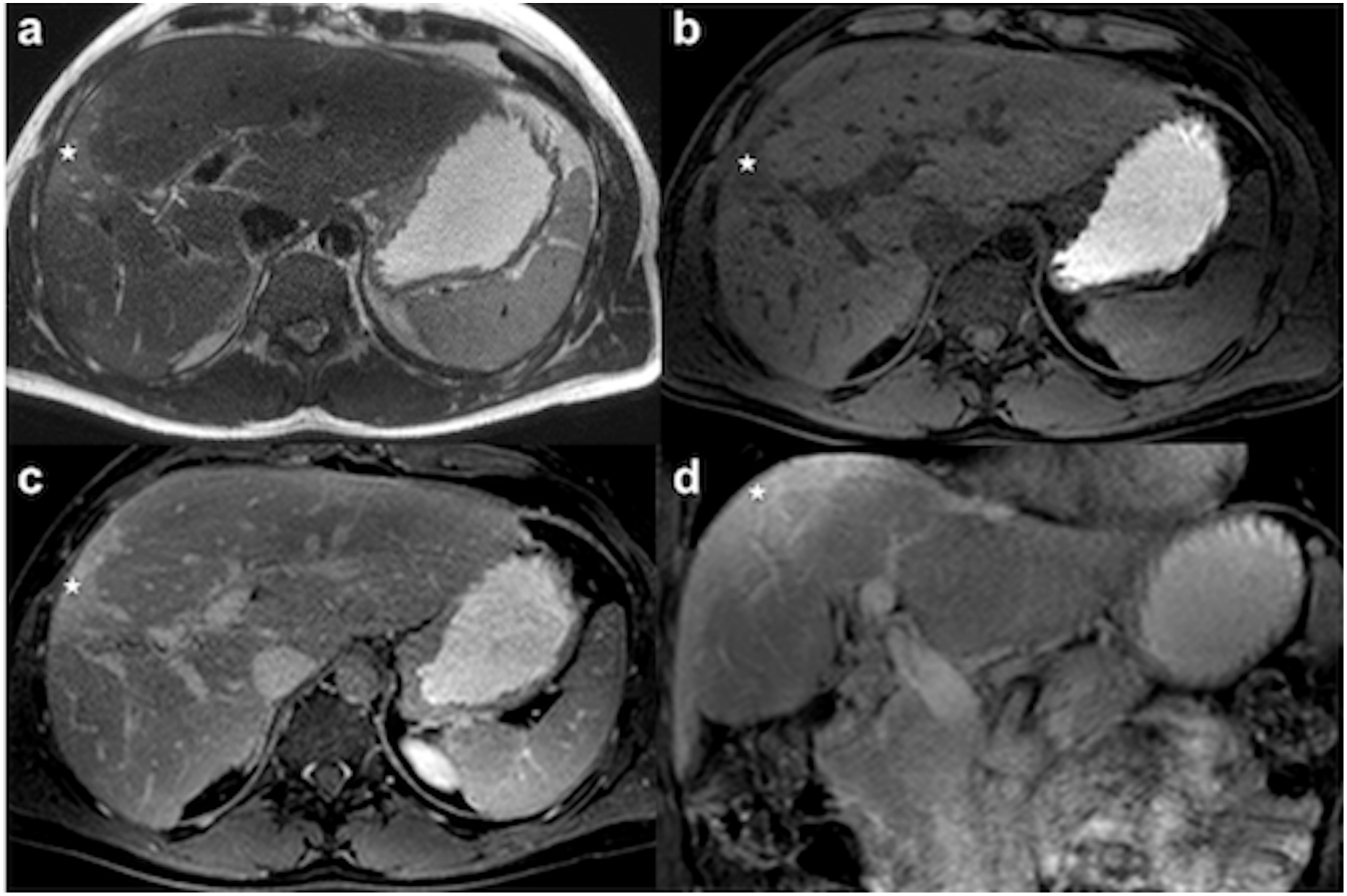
Wedge-shaped sub capsular area (asterisk) of T2 hyperintensity and GBCA accumulation on delayed phase imaging of a 39-year old male patient. (a) Axial T2-weighted image; (b) Non-enhanced axial T1-weighted fat suppressed image; (c, d) T1-weighted delayed phase axial and coronal image.
